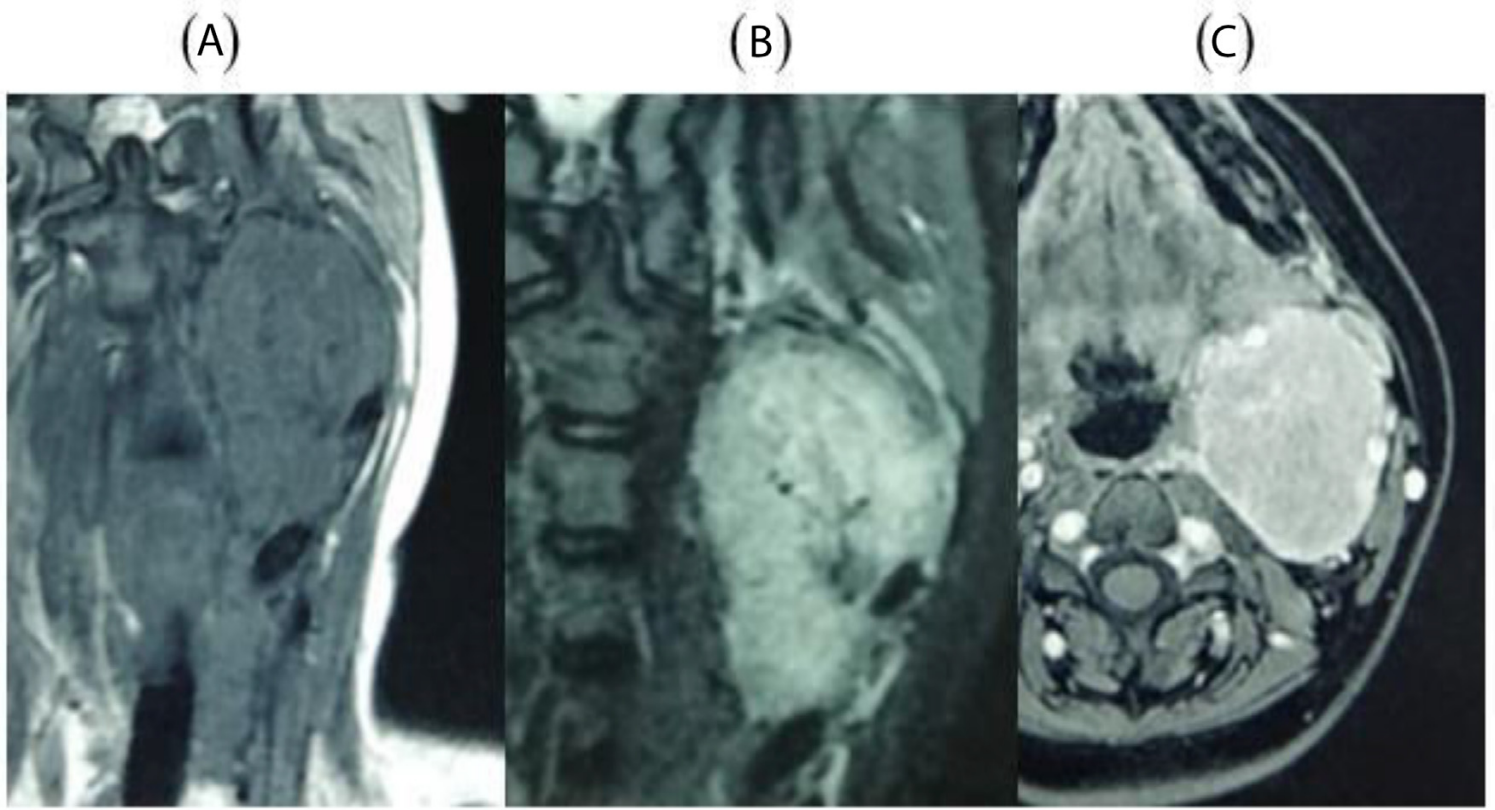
MRI: (A) Coronal T1-weighted: isointense mass; (B) Coronal T2-weighted: hyperintense mass; (C) Axial T1 post-gadolinium: avid enhancement. ICA and ECA are labeled.
